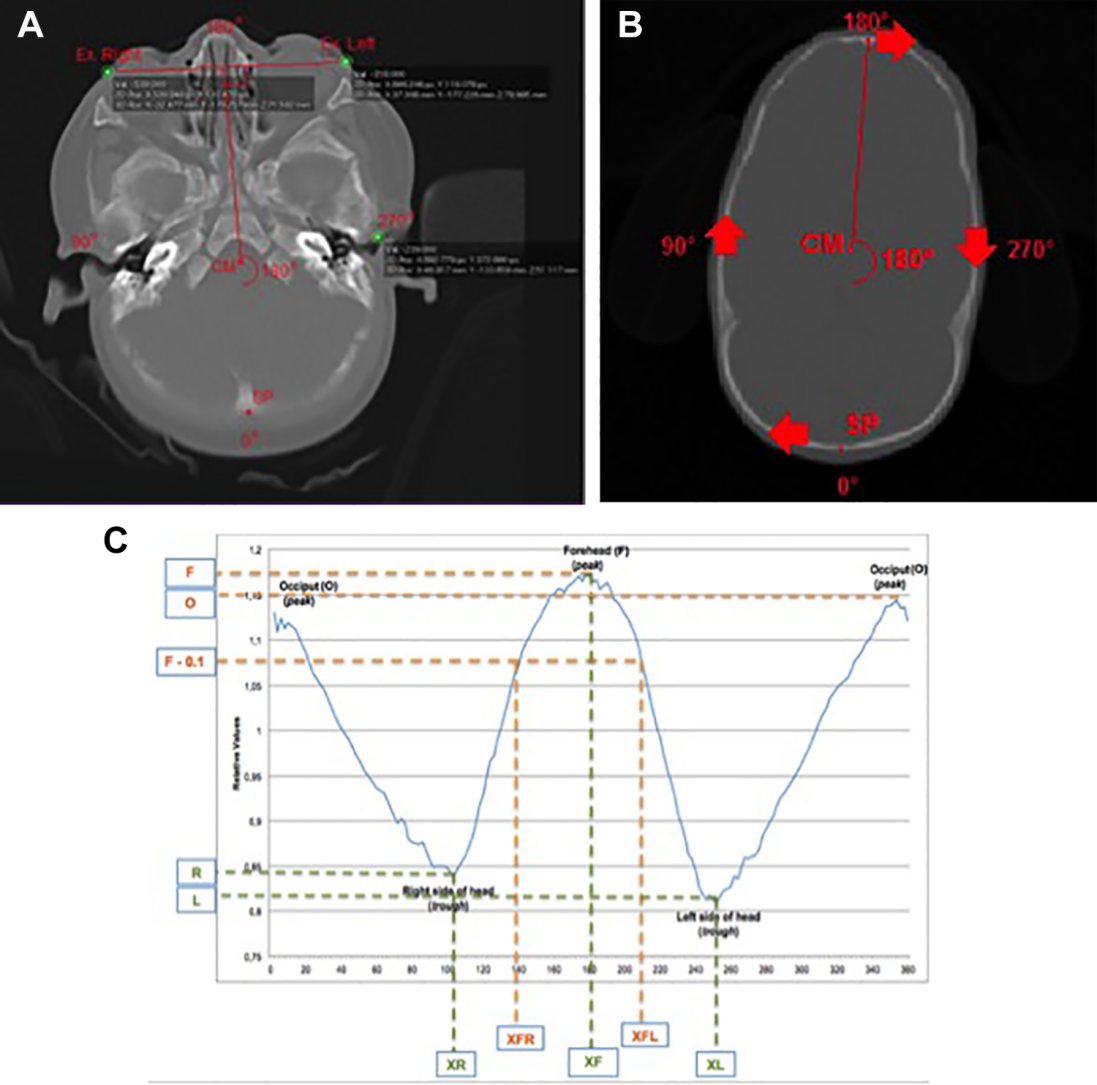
Visualization of the starting point of the curve and the resulting sinusoid curves and the used variables; the outline was made with the slices shown in (B). A, Plane on 0-cm height; this figure shows how the starting point of the curve is determined. Also, the degrees of the circle/outline are added, which are represented in the curve. B, Plane on 4-cm height; this figure shows the starting point and the direction of the curve. C, Shows the resulting curve; the different variables are marked. Curve starts at occiput (SP; B) and follows the skull outline (on CT scan) clockwise; therefore, first trough represents the right side of the head. Second peak is forehead; second trough is left side of the head. Curve stops where it started (at the occiput). CM, center of mass; F, maximum of forehead; L, minimum value of left side of head; O, maximum of occiput; R, minimum value of right side of head; SP, starting point; XF, X-value of maximum forehead value; XFL, X-value for the maximum forehead minus 0.1 on the left side; XFR, X-value for the maximum forehead minus 0.1 on the right side; XL, X-value of the minimum value of the width on the left side; XR, X-value of the minimum value of the width on the right side. CT indicates computed tomography.
